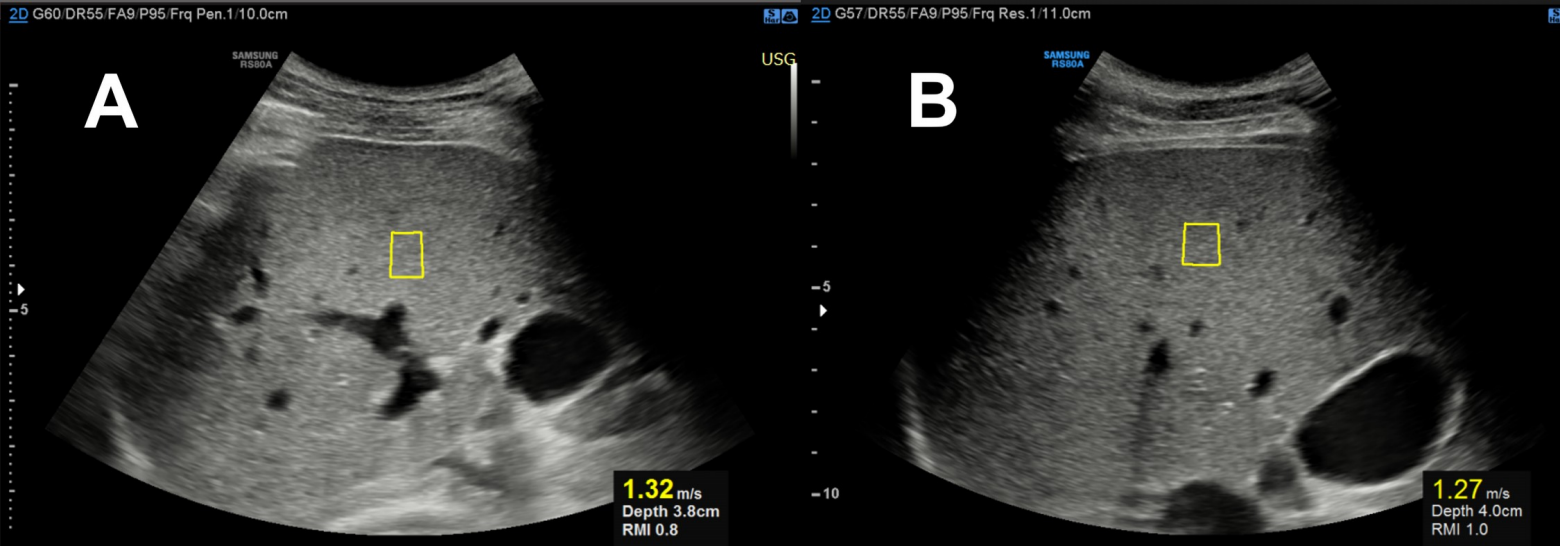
Right upper quadrant ultrasound of two patients using point shear-wave elastography technique. (A) A 48-year-old male patient, and (B), a 38-year-old male patient, both asymptomatic, 6 months after onset of severe yellow fever. Images depict placement of regions of interest (ROIs) on the right lobe of the liver at expiratory breath-hold. Shear-wave velocities (in m/s) were generated, with corresponding liver stiffness values after multiple measurements of 5.3 kPa and 4.6 kPa, respectively. Both subjects were then classified as F0/F1 on the Metavir scoring system for staging of fibrosis. RMI = Reliability measurement index.
